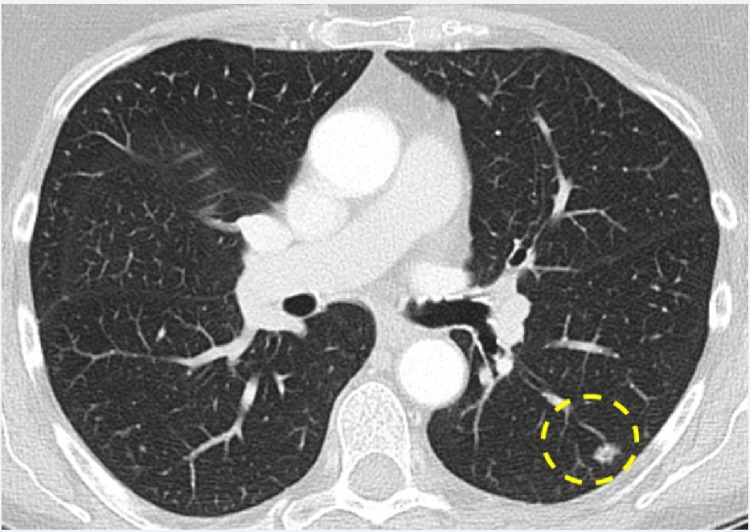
Enhanced CT of the chest with lung algorithm Axial image through the level of the left lower lobe nodule (perforated yellow circle). Note: patient in supine position.
